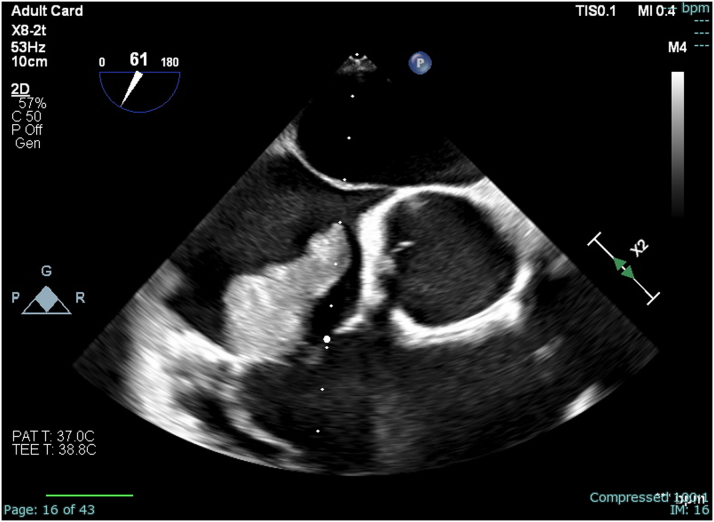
Large Vegetation (>3 cm) Present on the Tricuspid Valve on Midesophageal Right Ventricular Inflow-Outflow Preprocedural Transesophageal Echocardiography
